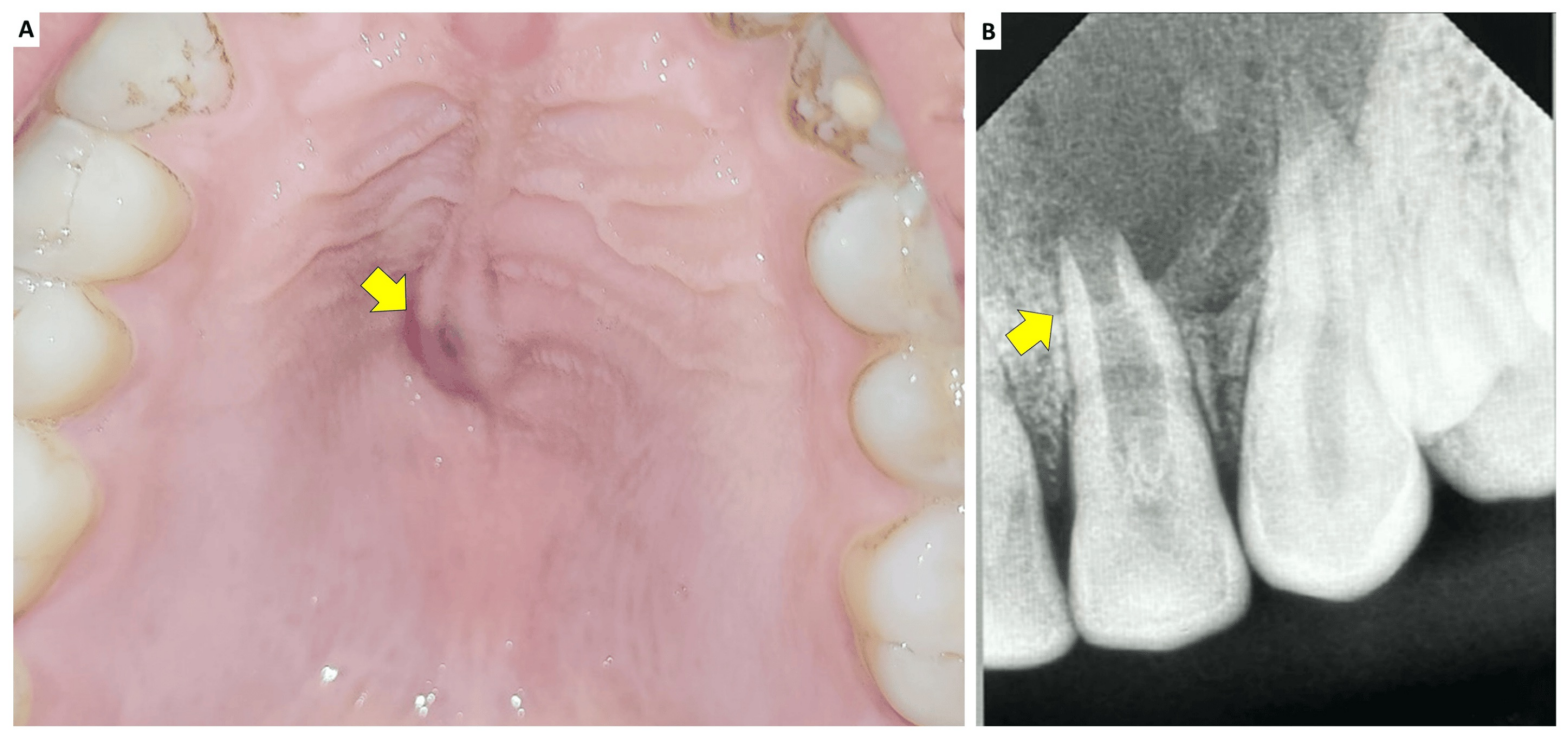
(A) Preoperative images of #22 with palatal non-draining sinus and (B) periapical radiography of tooth #22 showed a radiolucent pocket with a radiopaque border extending into the root as a blind sac up to the cementoenamel junction. A blunderbuss canal #22 and a well-defined periapical radiolucency involving the apices of teeth #21, #22, and #23 were evident
